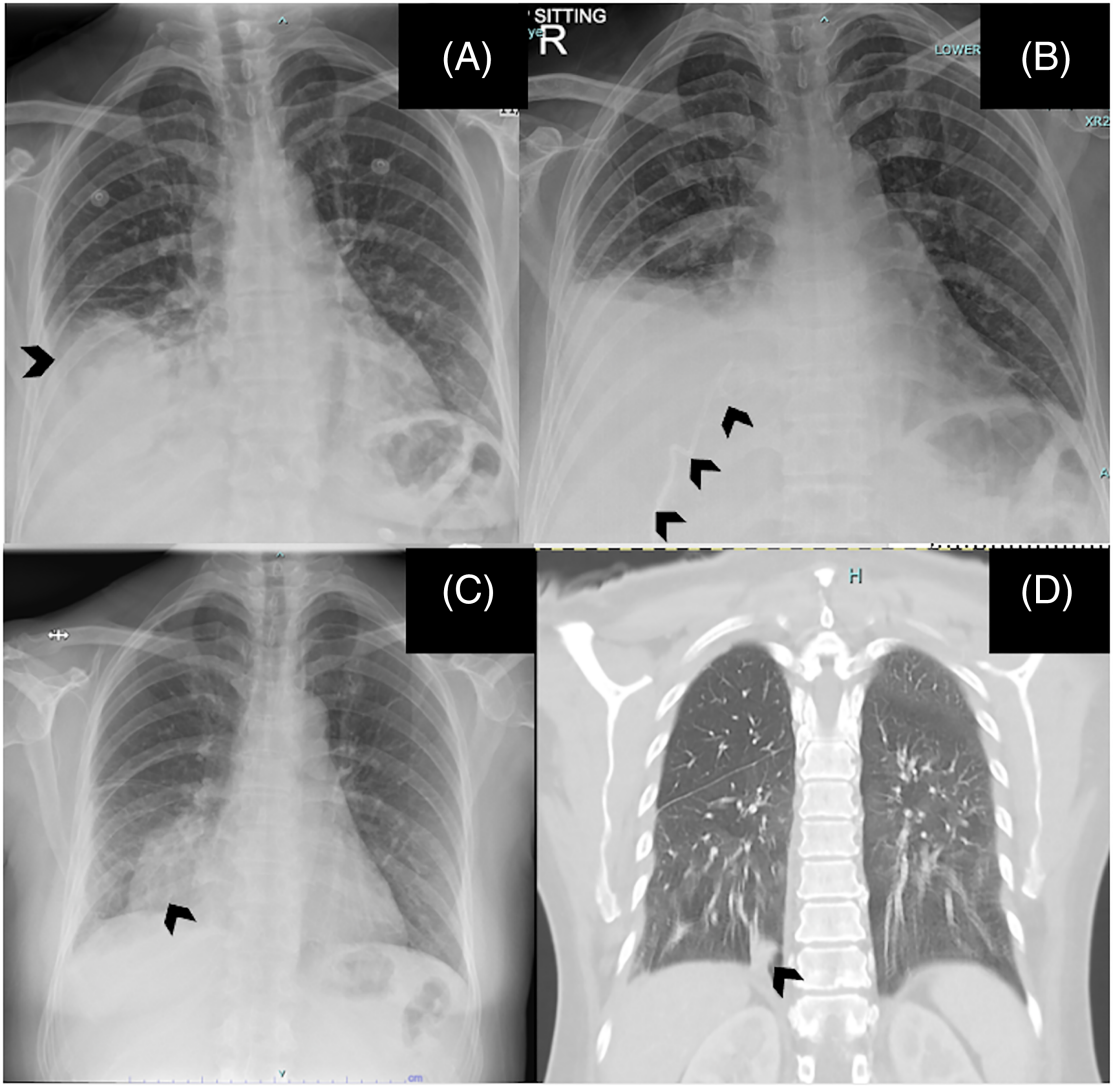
(A) Chest radiograph revealed right lower zone opacity with blunting of costophrenic angle. (B) Chest radiograph showed ICC in‐situ (arrow) with persistent pleural effusion. (C) Chest radiograph showed significant improvement of right pleural effusion after completion of intrapleural therapy; with presence of atelectatic lung (arrow). (D) CECT Thorax on follow‐up showed resolution of effusion with rounded atelectasis right lung (arrow).
